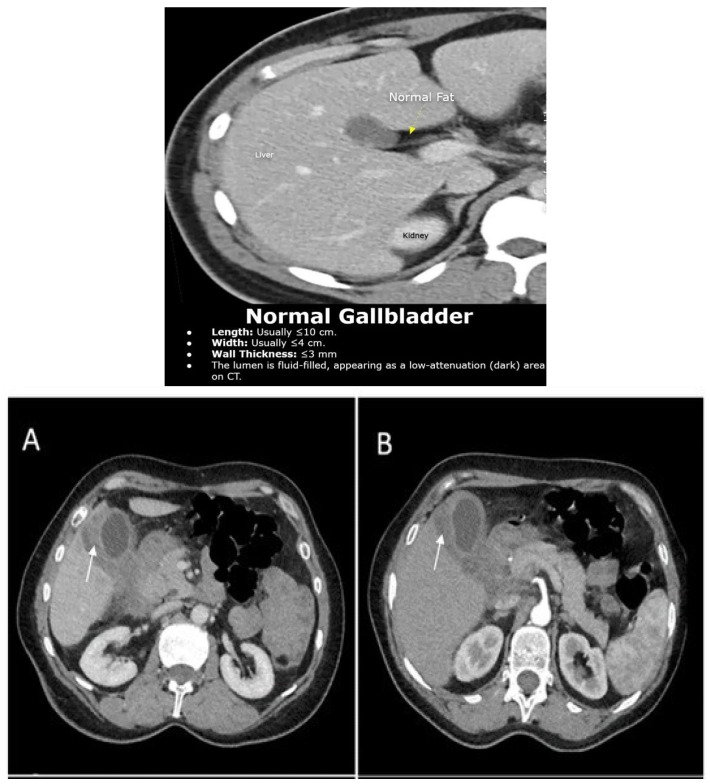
Contrast-Enhanced CT Imaging of the Gallbladder Left: Normal gallbladder aspect (Upper). Panels (A,B): Gallbladder with irregular mural thickening (white arrow), associated pericholecystic fluid, and loco-regional inflammatory lymphadenopathy.
